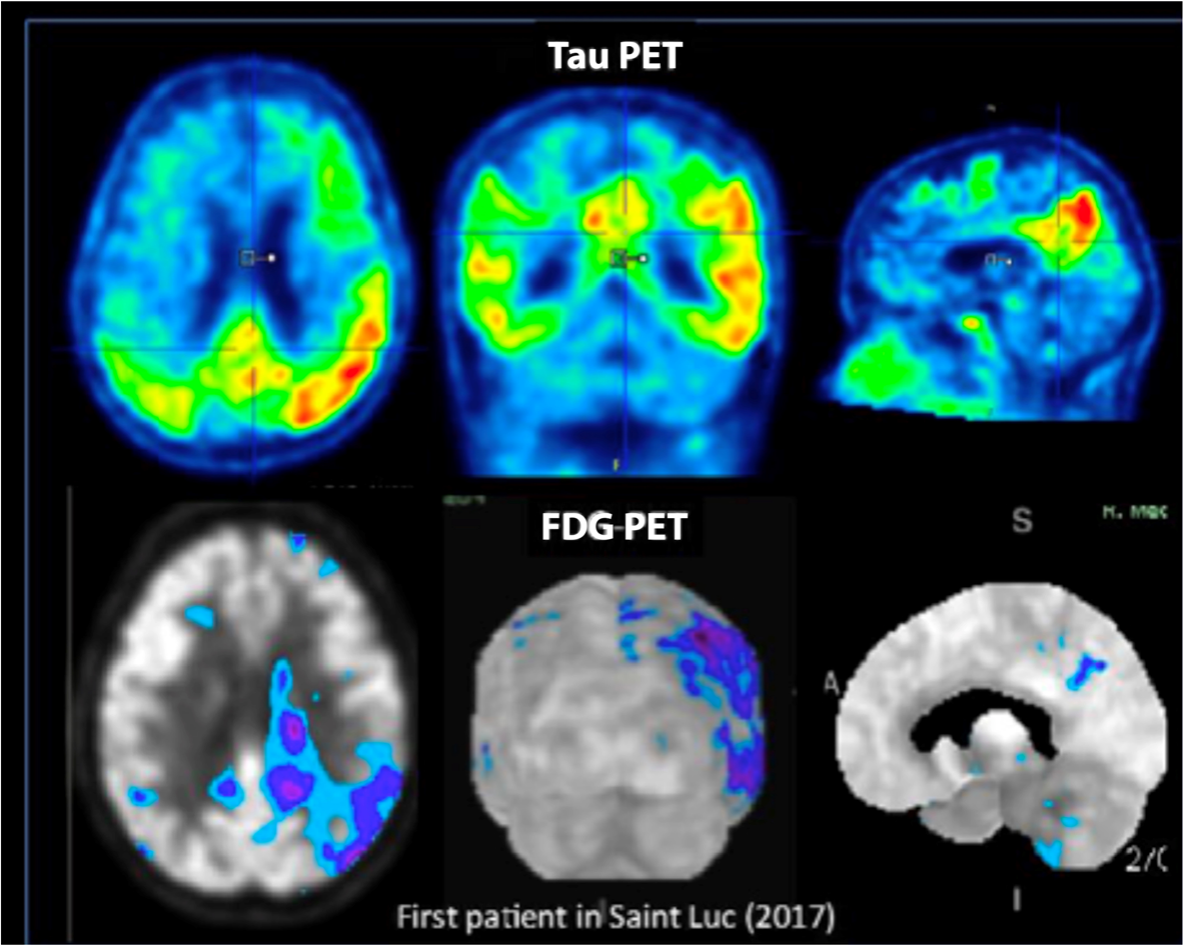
Tau PET image in a patient with AD ( Mini Mental State Examination= 20/30), demonstrating close association between tau pathology (top) and cerebral glucose metabolism (FDG-PET, bottom). Images were acquired at Saint-Luc University Hospital (UCLouvain, Belgium)
